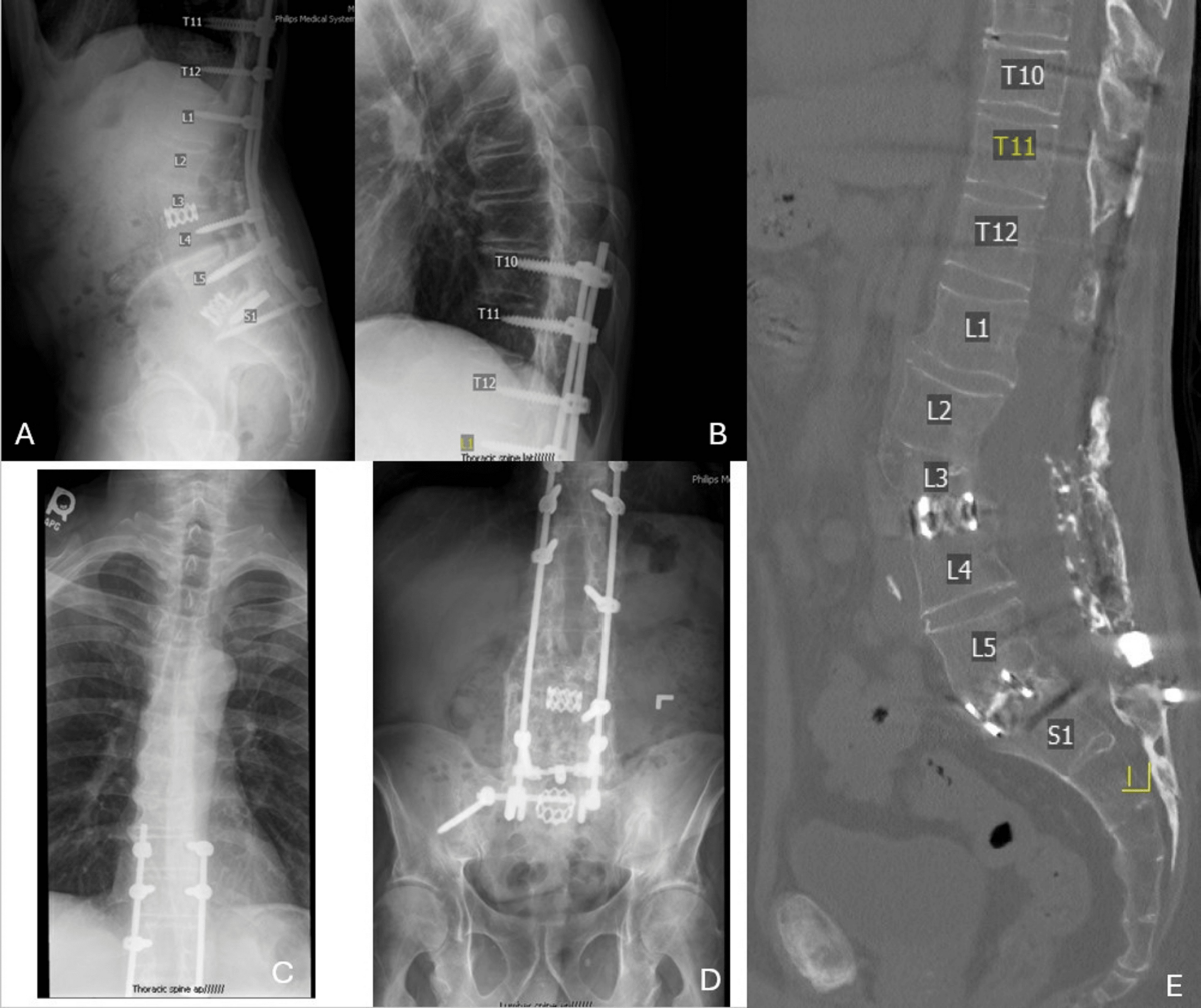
Preoperative lumbar images showing initial construct after 10 revisions A: preoperative lateral lumbar radiograph, B: preoperative lateral thoracic radiograph, C: preoperative AP thoracic radiograph, D: preoperative AP lumbar radiograph, E: sagittal lumbar CT AP: anteroposterior, CT: computed tomography
